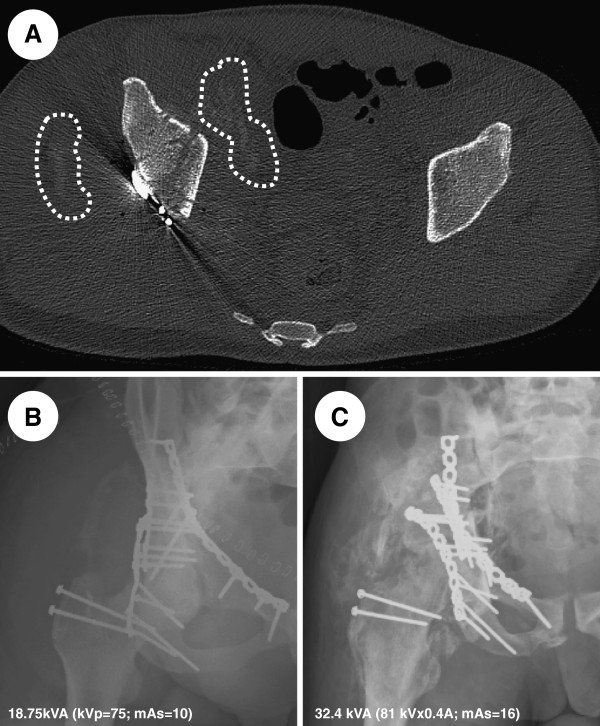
Early and late radiological evidence of heterotopic ossification around the right hip. (A) Axial computerized tomography (CT) scan cut of the acetabular region 11 days post-trauma. Note the denser soft tissues (encircled) surrounding the right fractured acetabulum. Radiographic AP view of the right hip at 14 days; (B) and three and a half months; (C) post-trauma. Note the progression of calcified soft tissues medially and laterally within the abductor muscles.
